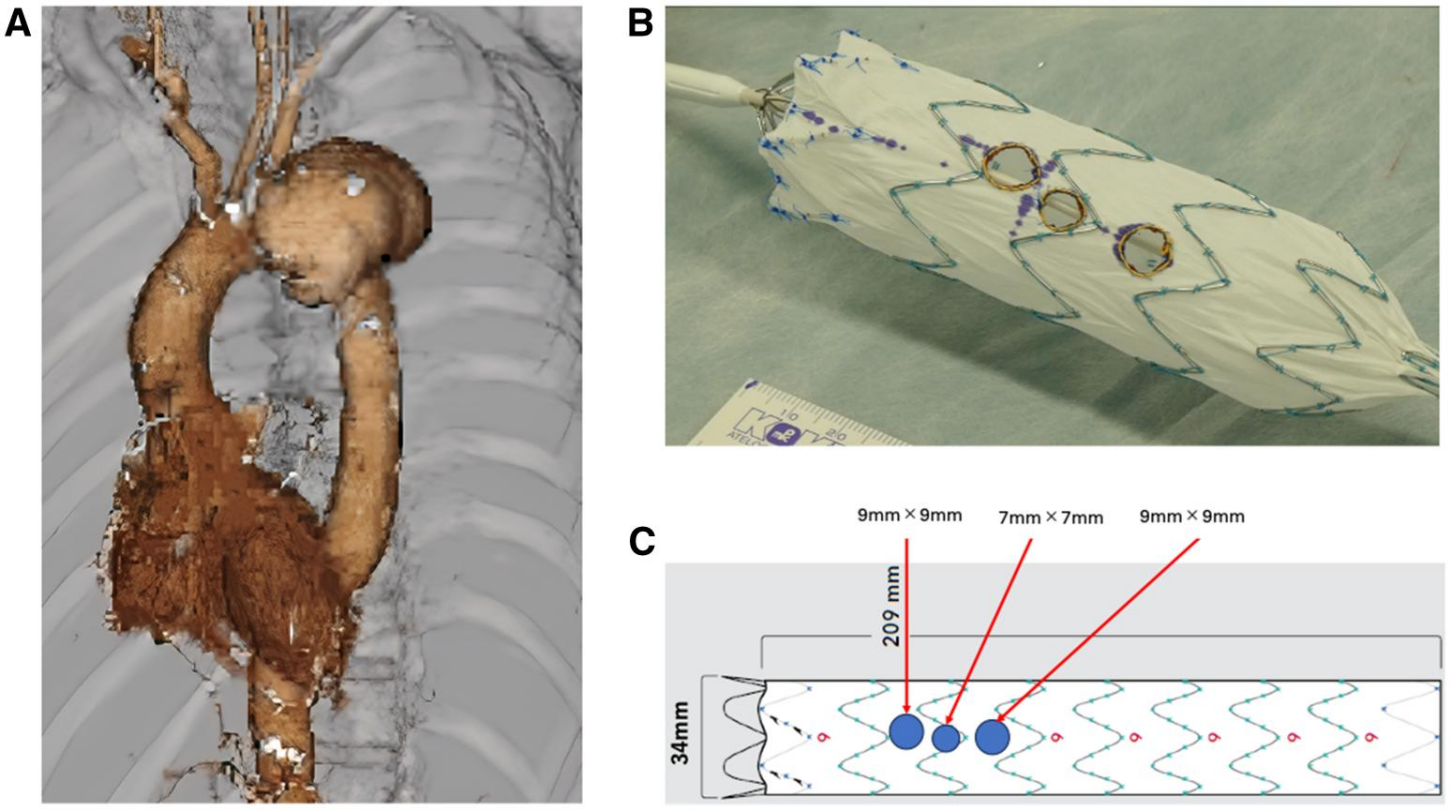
Preoperative Imaging and Fenestrated Stent-Graft Preparation. (A) Preoperative aortic computed tomography angiogram showing a dissecting aortic aneurysm in the arch. (B, C) Three fenestrations (brachiocephalic artery: 9 × 9 mm, left common carotid artery: 7 × 7 mm, and left subclavian artery: 9 × 9 mm) were created on the stent-graft using high-temperature cautery
